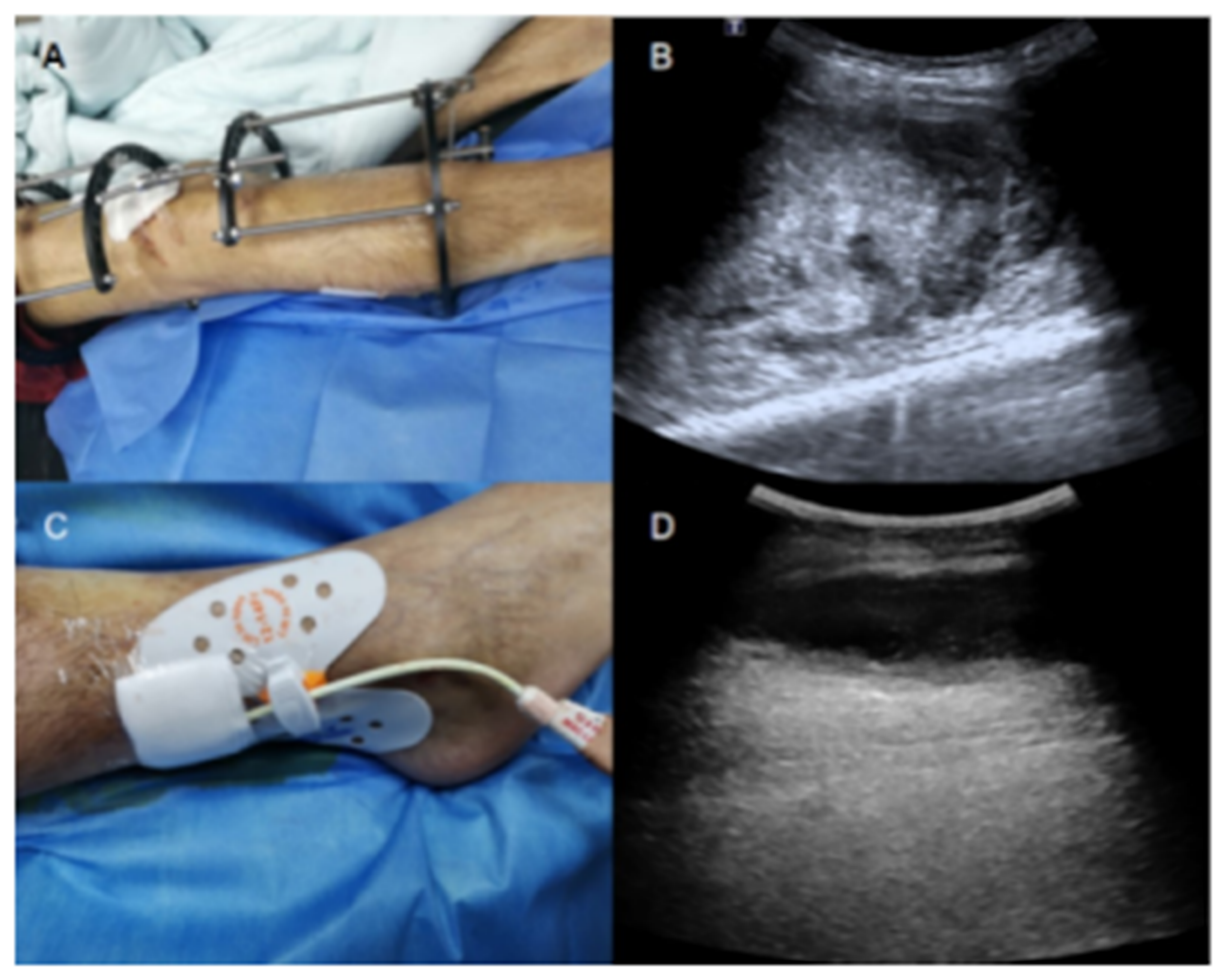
Ultrasound examination revealed a polycystic hematoma in the forearm flexors (long axis section of the hematoma; (A). The hematoma was located between the median nerve (*), and radius-ulna (short axis section of the hematoma; (B). Three days after drainage and urokinase lavage, ultrasonography showed that the hematoma nearly disappeared (C). Hematoma did not recur at the 4-month follow-up after treatment (D).
